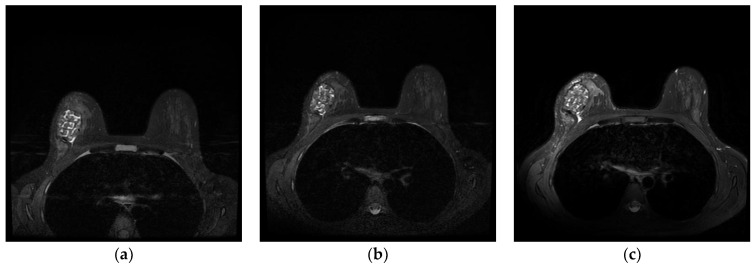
Serial magnetic resonance imaging demonstrating long-term volume stability of diced ADM with paste-type ADM in a 40-year-old patient who underwent oncoplastic breast-conserving surgery. T2-weighted axial images show consistent low signal intensity of the ADM construct at (a) 6 months postoperatively, (b) 12 months postoperatively, and (c) 24 months postoperatively. Quantitative analysis revealed minimal volume reduction (3.7%) over the 24-month follow-up period, confirming the long-term stability of the ADM reconstruction. Note the well-defined margins and homogeneous signal characteristics of the ADM throughout the follow-up period, indicating successful tissue integration without significant degradation or resorption.
